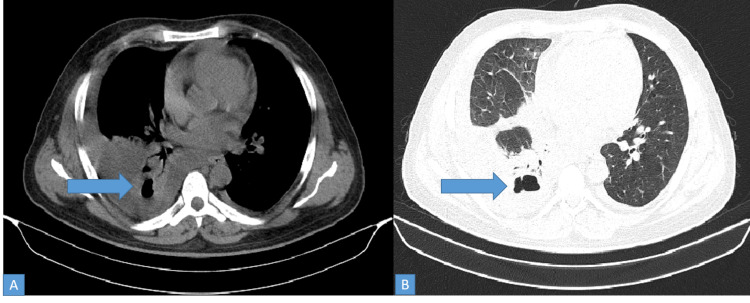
CT thorax showing collapse, consolidation with cavitation in right lower lobe (A-bone and B-lung windows)
